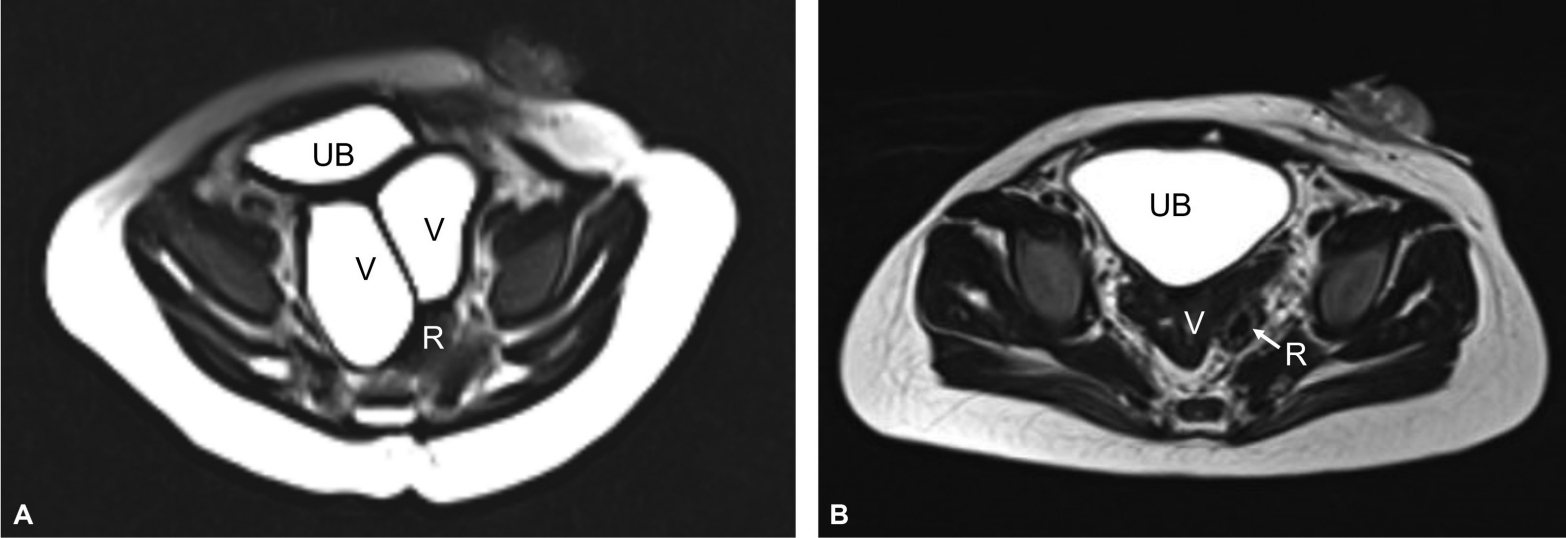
Pelvic magnetic resonance imaging (MRI) (axial T2-weighted image [T2WI]) of the third case comparing vaginal distension before (
